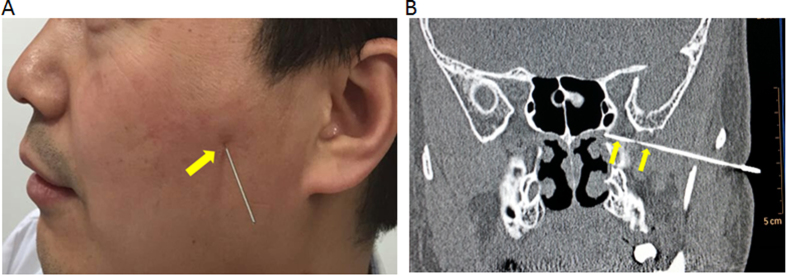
(A) Site of acupuncture in sphenopalatine ganglion(yellow arrow); (B) High resolution CT scan of pterygopalatine fossa in coronal plane after the acupuncture showing needle penetration intopterygopalatine fossa(yellow arrows).
